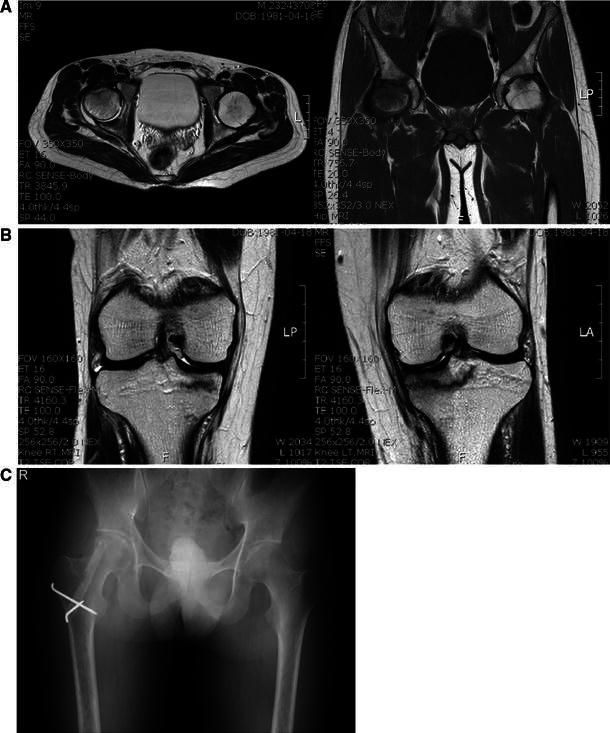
a The magnetic resonance imaging (MRI) obtained showed diffuse signal intensity (SI) decreases across the entire right head of the femur. b The magnetic resonance imaging (MRI) obtained showed that subchondral zones of low SI were observed in medial tibial plateaus on both sides. c Close reductions and internal fixations with fibular shaft graft and s-pins were performed on the subchondral stress fractures. At 2 years postoperatively, the patient still had considerable depressed deformation in the right femoral head
